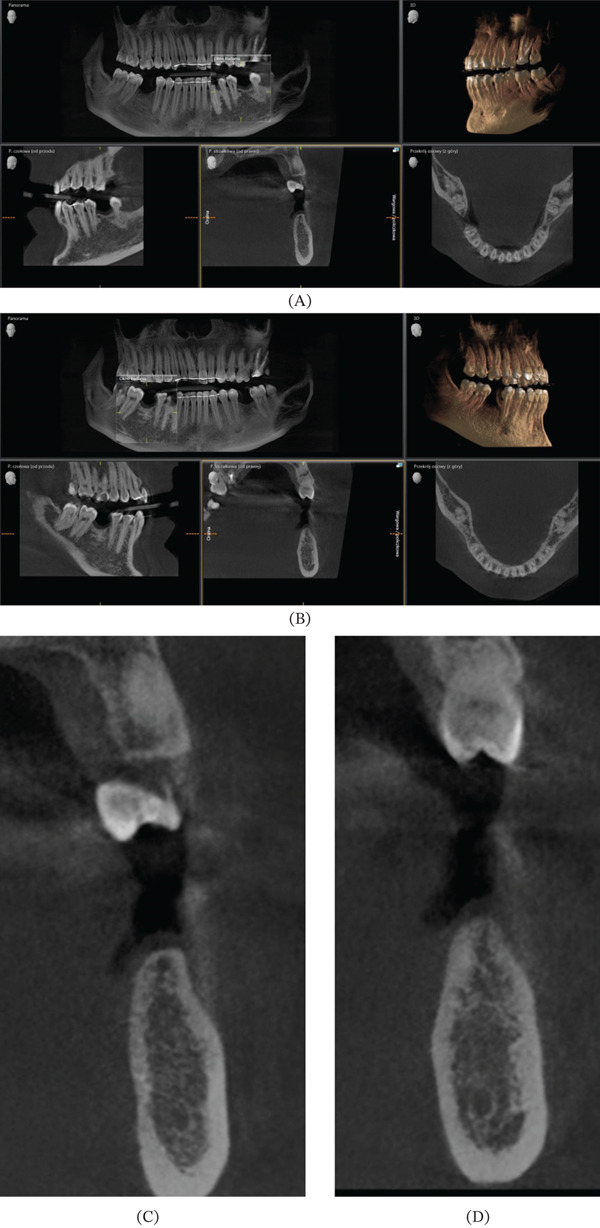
Radiological diagnostics prior to treatment based on CBCT examination. (A) CBCT evaluation of the left posterior mandibular region, (B) CBCT evaluation of the right posterior mandibular region, (C) cross‐section—left posterior mandible, and (D) cross‐section—right posterior mandible.
